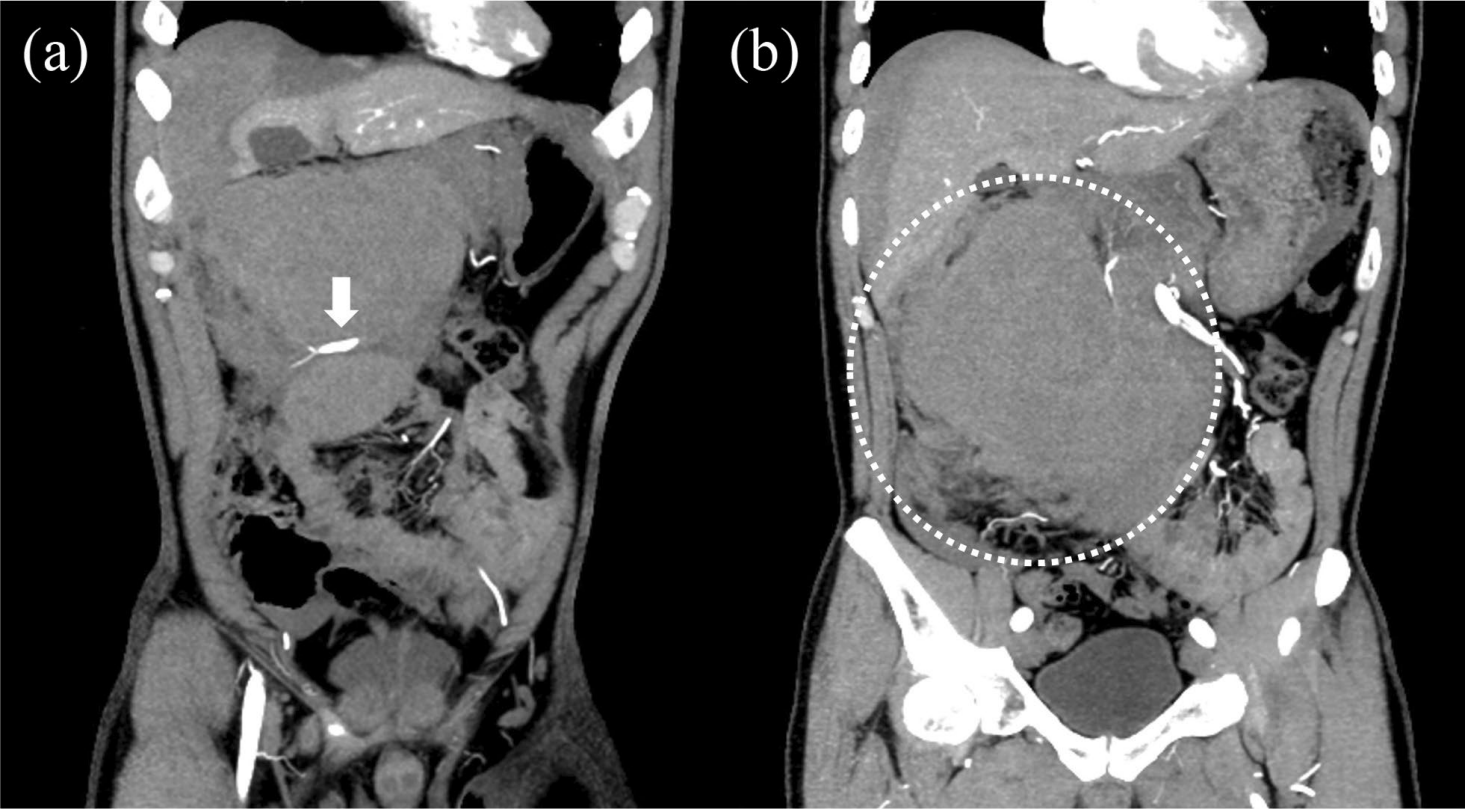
The computed tomography image shows the spindle-shaped aneurysm of the right colic artery (arrow) (a) and a large mesenteric hematoma (b)
